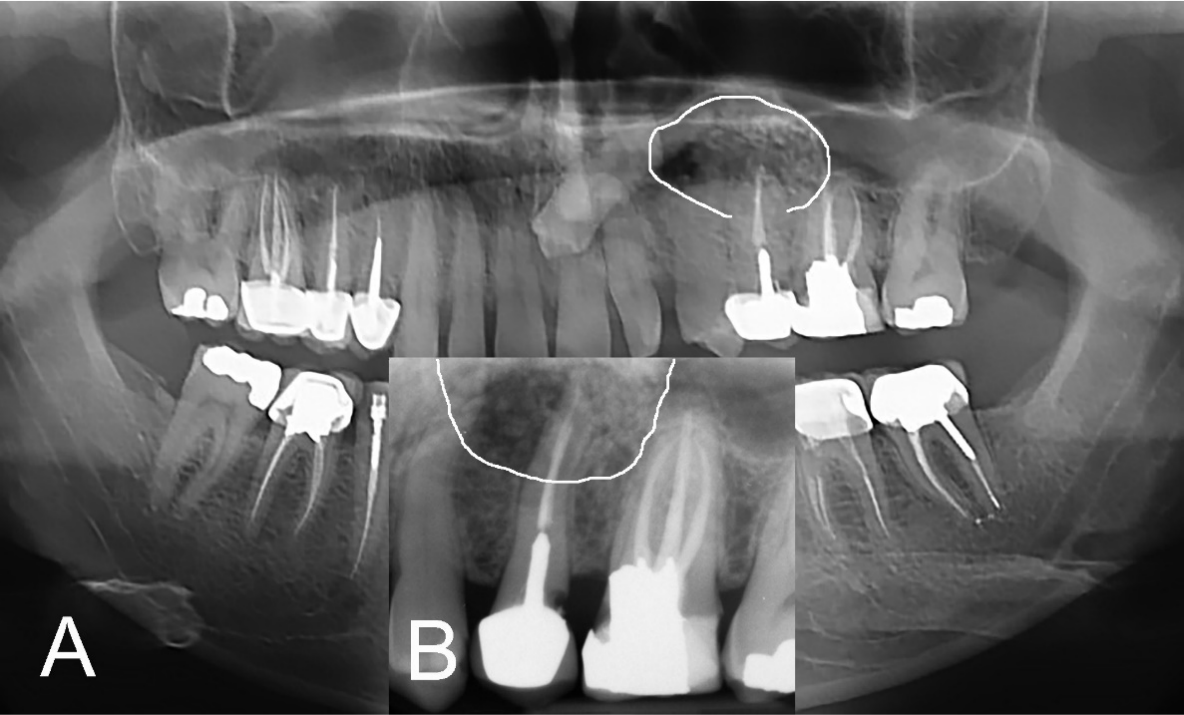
Primary radiographic evaluation, diffuse regenerative materials and unhealed periradicular lesion (white lines) around the endodontically treated root of the maxillary left second premolar; *A)* Panoramic radiograph, and *B)* Periapical radiograph
